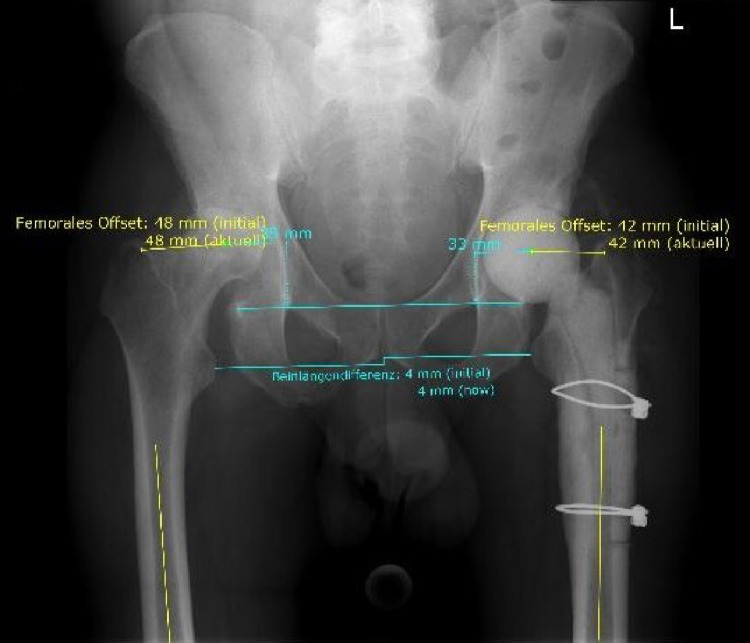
Radiographs with measurement of the femoral offset and leg length
